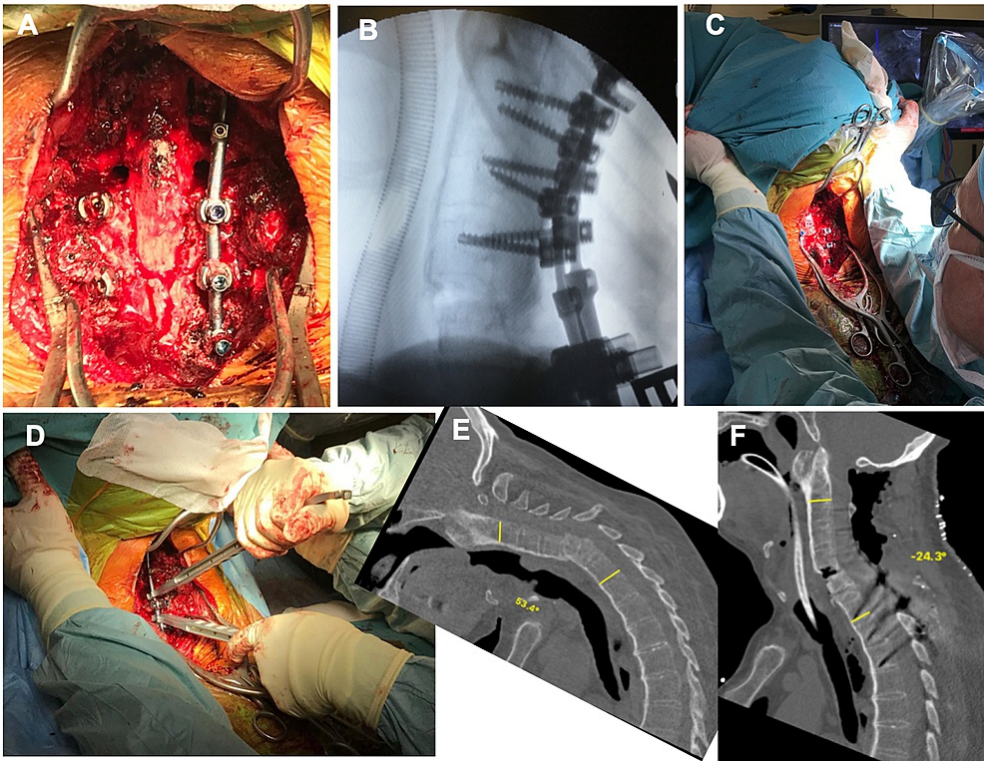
A) Wide laminectomy with C7 PSO performed and C7 and C8 roots decompressed before closure; B) Image from intraoperative fluoroscopy after osteotomy closure. Opening of the anterior column at the fracture site attained during primary closed reduction; C) Closing of the osteotomy site along with head repositioning. D) in situ rod bending E) preop CT scan with 53.4 of cervical kyphosis and F) postop CT scans showing 24.3 degrees of lordosis.
